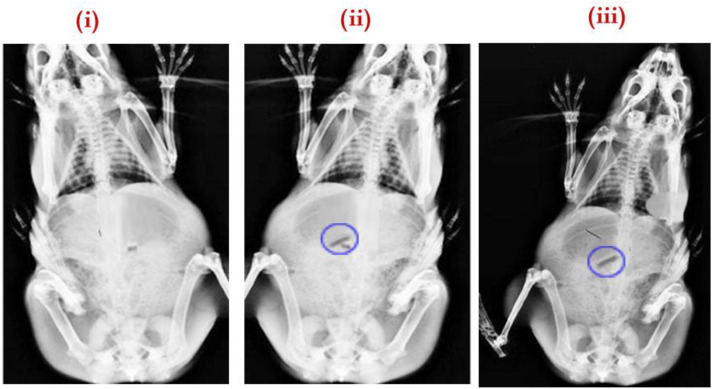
X-ray radiographs (i) before administration and after the administration of O-IH-UF at (ii) 1 h and (iii) 8 h.
